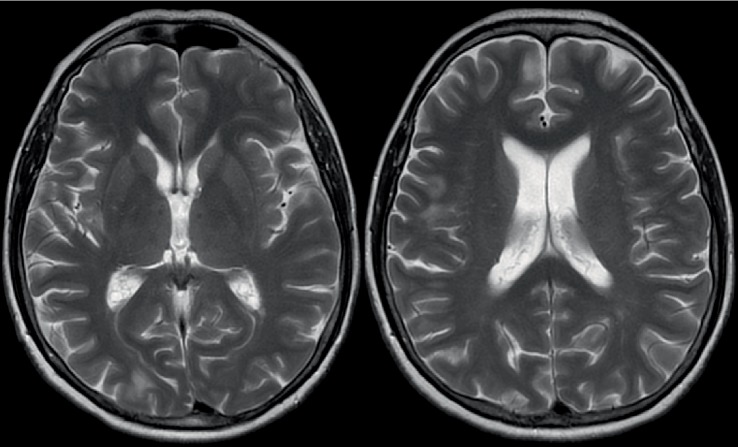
Transversal T2-weighted MR images one year after endovascular treatment revealed only subtle remaining signal changes.
